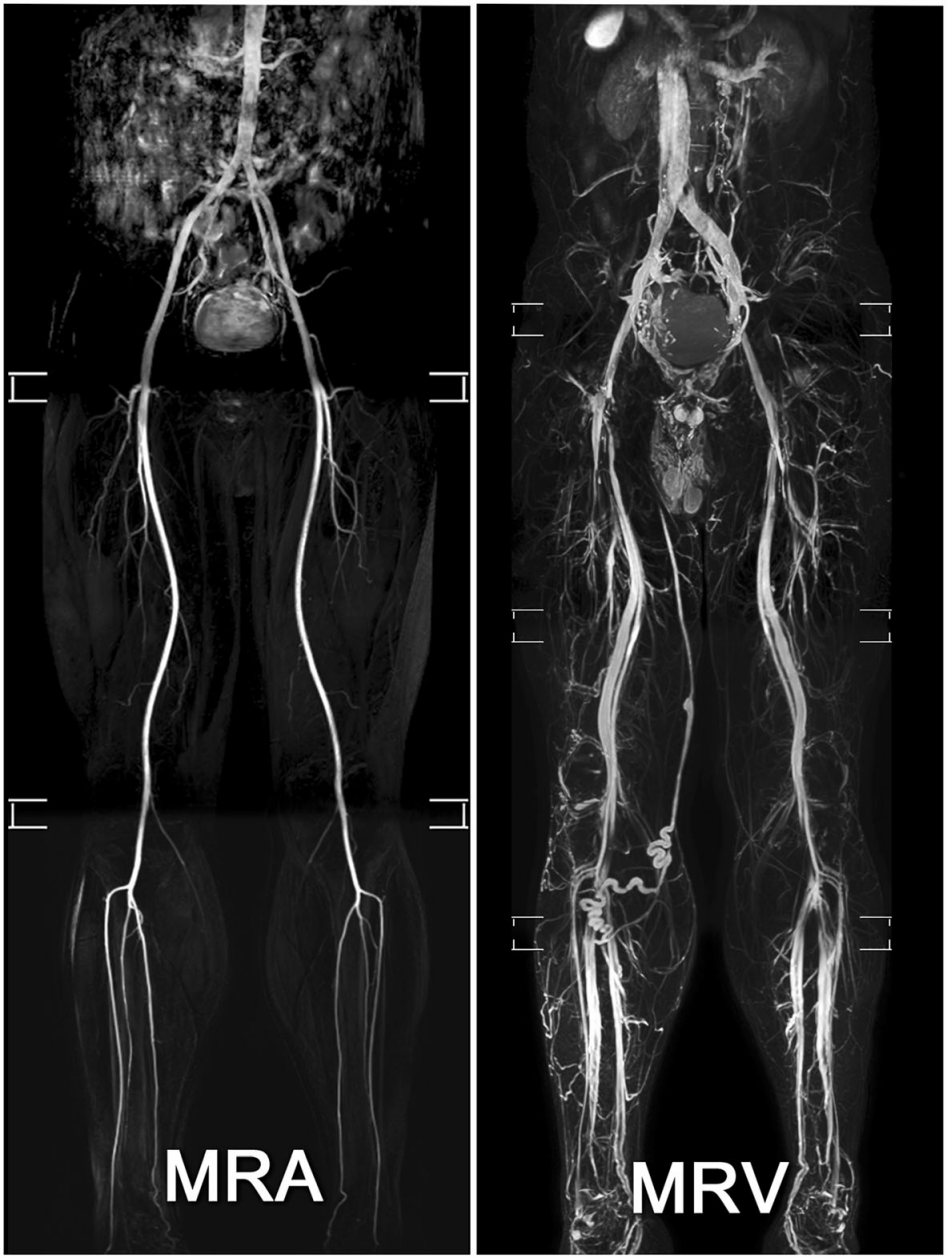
TRANCE MRA and MRV images, TRANCE-MRI was performed on an elder patient. MRA showing arterial patency of bilateral lower extremities. MRV showing a large varicose vein along the right greater saphenous vein
